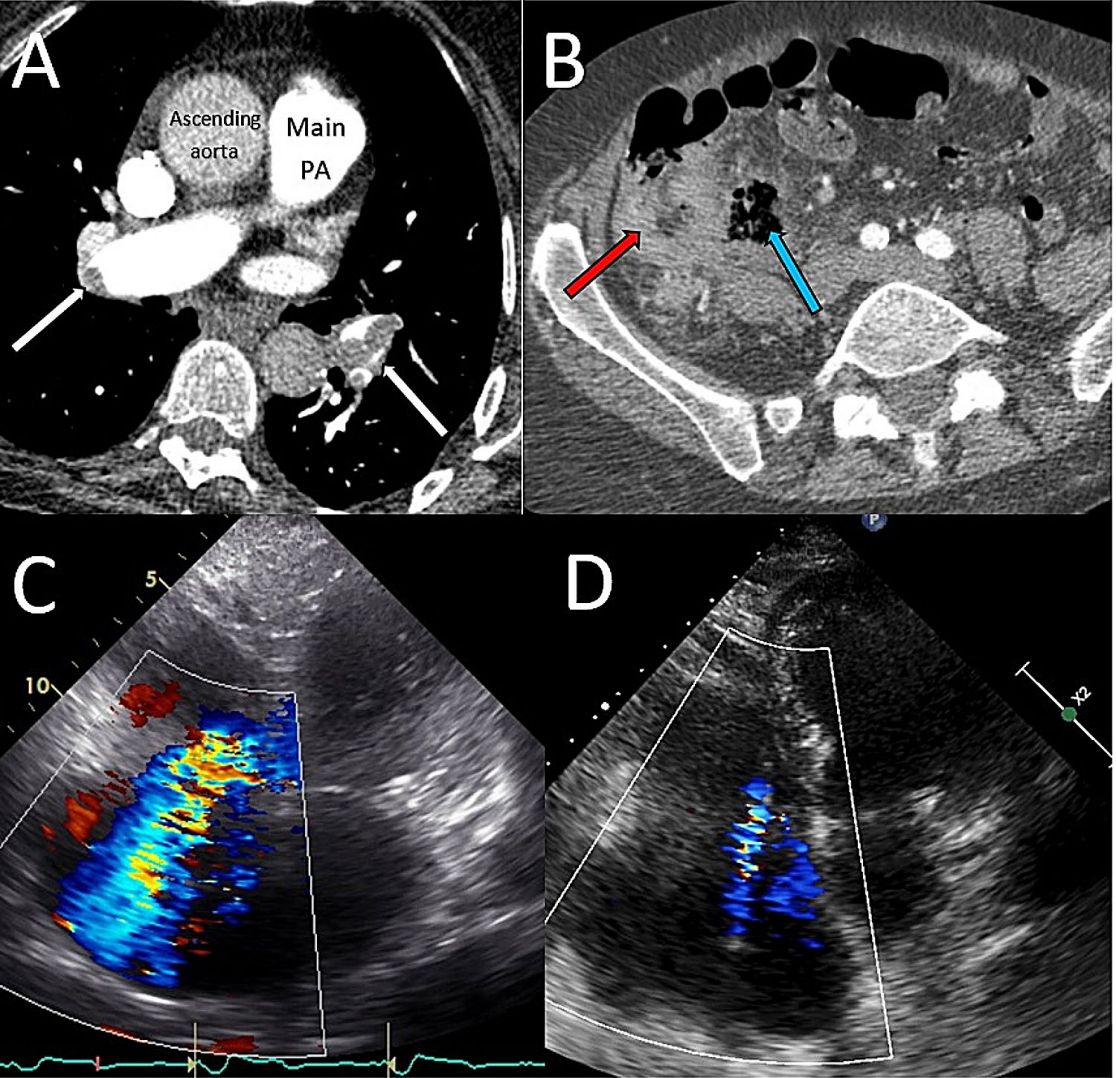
a CTPA showing bilateral PE, b CT abdomen showing marked caecal thickening (red arrow) and adjacent air pocket secondary to bowel perforation (blue arrow), c and d degree of TR pre, and post U-ACD
